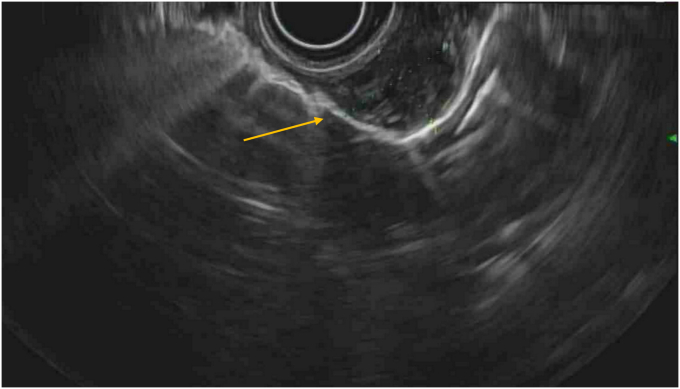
Endoscopic ultrasound image showing an 18- × 12-mm hypoechoic mildly heterogenous mass likely arising from the submucosa (*yellow arrow*).
